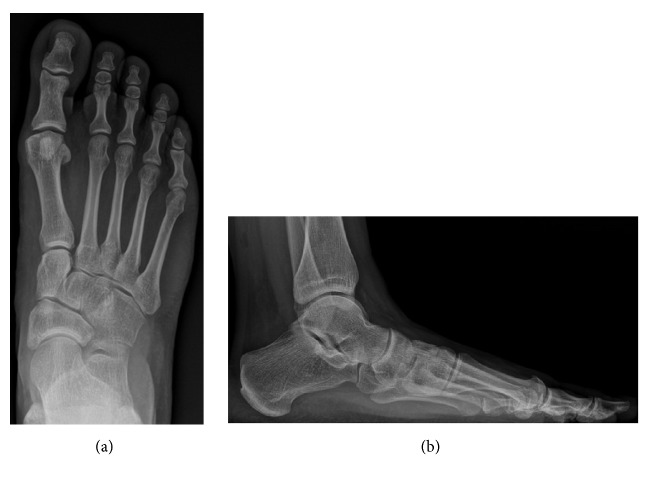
Preoperative anterior-posterior (a) and lateral (b) weight-bearing radiographs.
